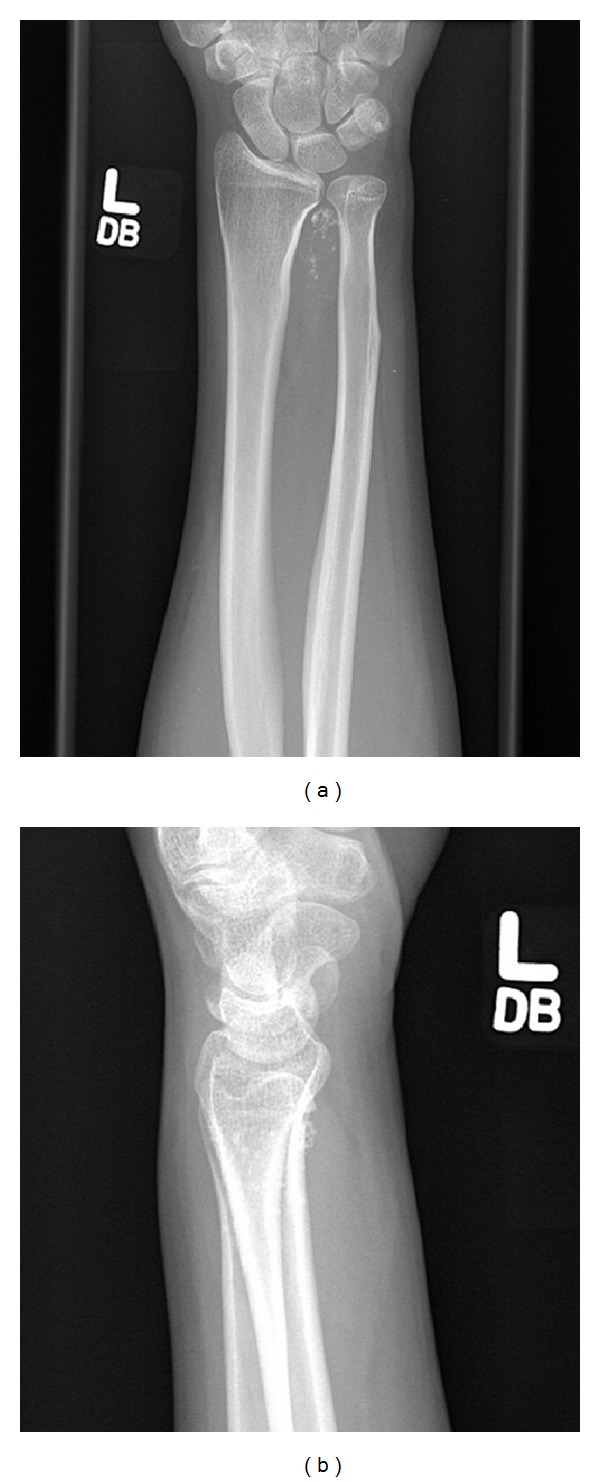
(a) and (b) PA and lateral X-rays of the left forearm and wrist showing calcified nodules consistent with synovial chondromatosis. Superficial cortical scalloping of the distal radius and ulna is noted.
